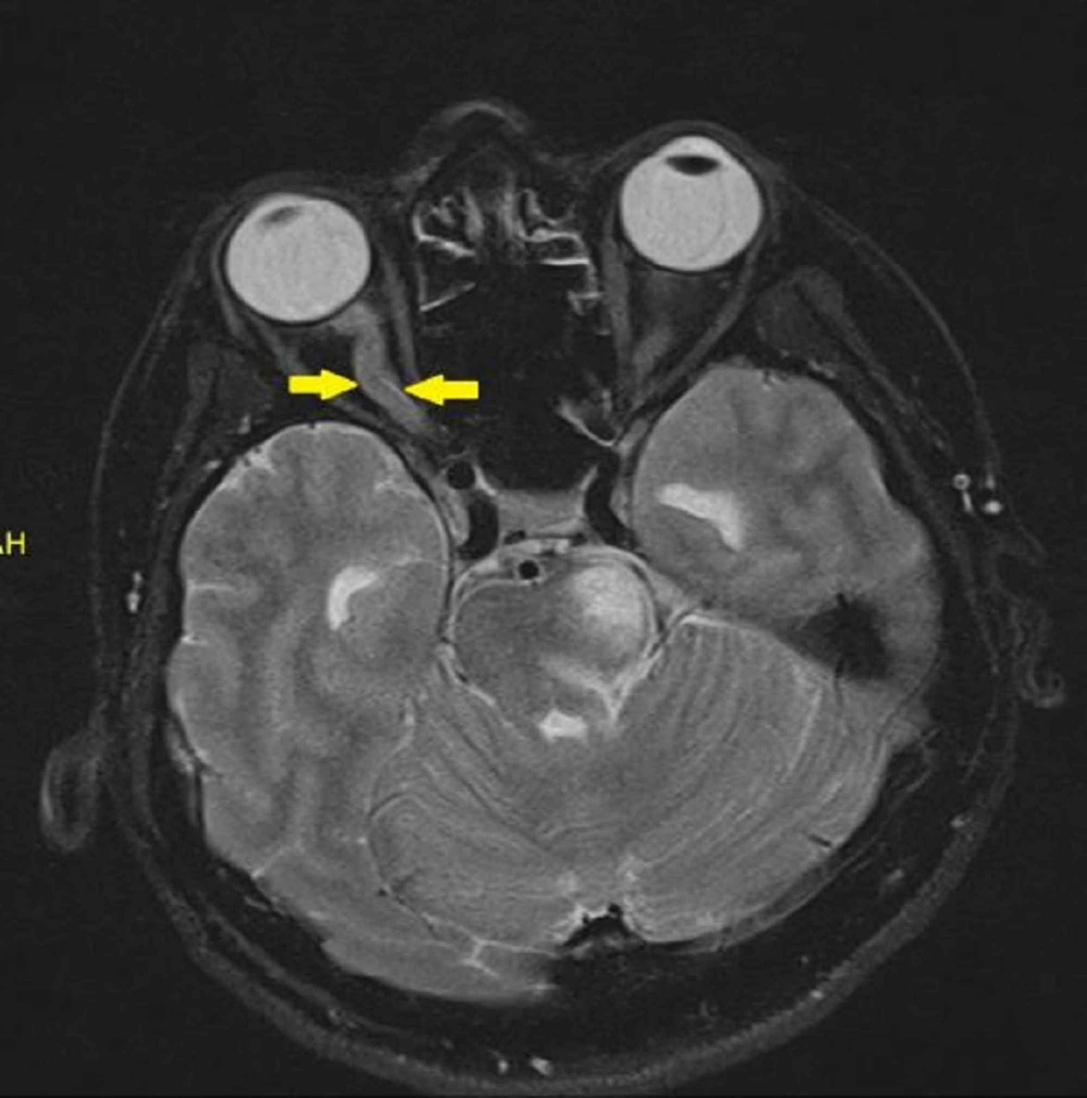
Magnetic resonance imaging (MRI) showed kinked right optic nerve with thickened optic nerve sheath
